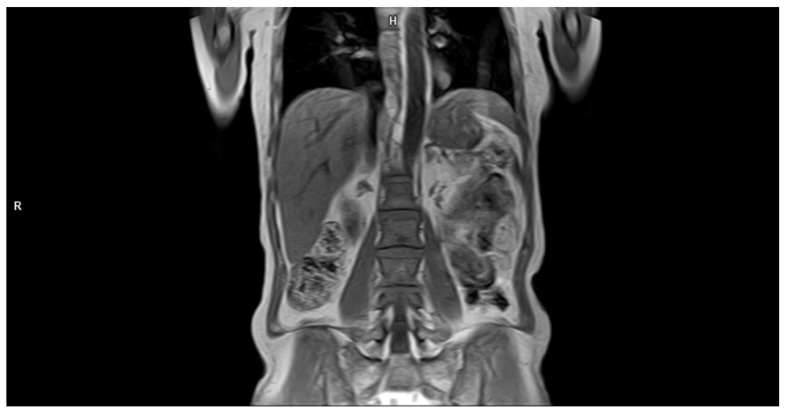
Coronal T1-weighted image of a spine demonstrating a “salt-and-pepper” pattern of marrow involvement in a 63-year-old woman with a multiple myeloma diagnosis. Source: Radiological Diagnostics Center, The National Institute of Medicine of the Ministry of Interior and Administration, Warsaw, Poland.
